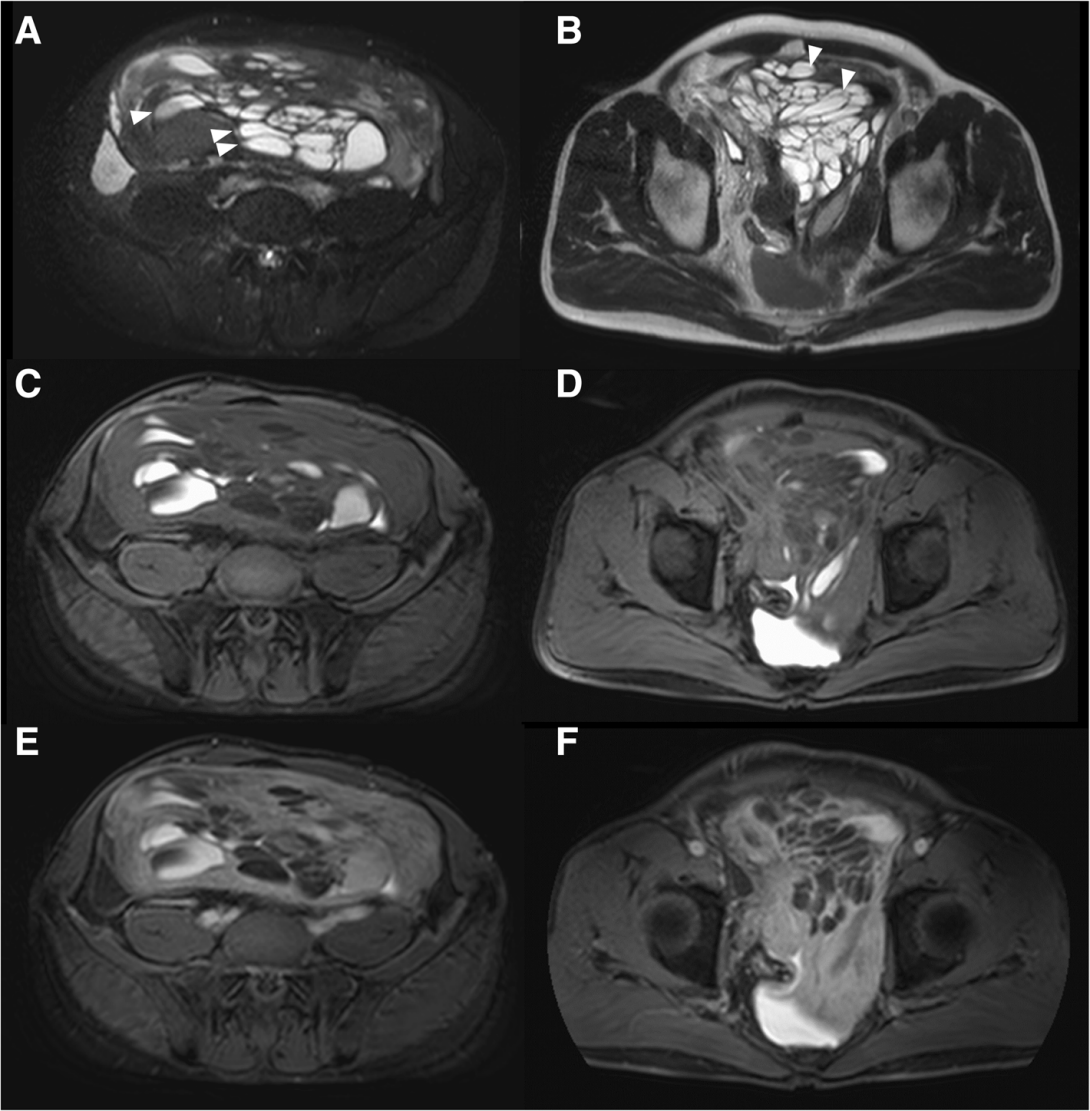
a, b T2-weighted MRI. Most cysts showed high intensity suggesting simple fluid, and some showed the presence of layering which suggests the likelihood of either fat or blood in content. The arrowheads show the presence of layering within the cystic areas. c, d Non-enhanced fat-saturated T1-weighted MRI and e, f gadolinium-enhanced fat-saturated T1-weighted MRI. Several solid components were enhanced
